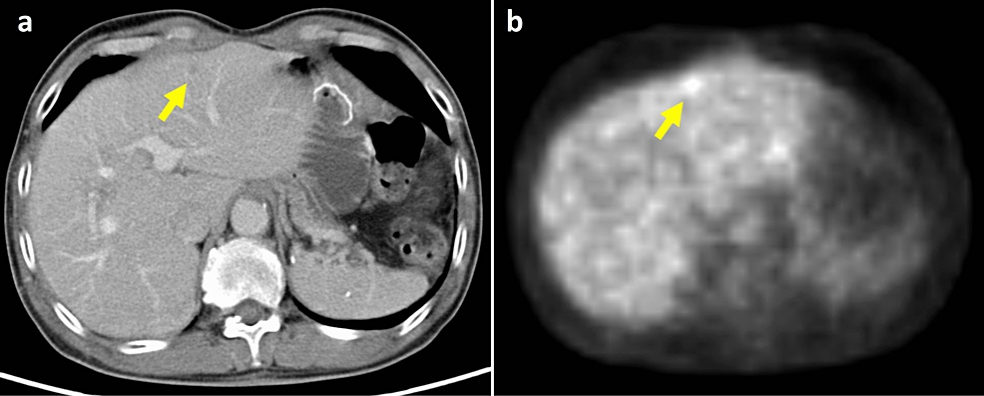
Contrast-enhanced CT and PET-CT one year after the first proton beam therapy. Contrast-enhanced CT (a) and PET-CT (b) one year after the first PBT. The liver metastasis was significantly regressed after four courses of docetaxel (70 mg/m^2^), cisplatin (70 mg/m^2^), and 5-FU (700 mg/m^2^) (yellow arrow). We could not rule out the possibility of metastasis from the EG junction cancer. EG: esophagogastric; PBT: proton beam therapy; 5-FU: 5-fluorouracil
